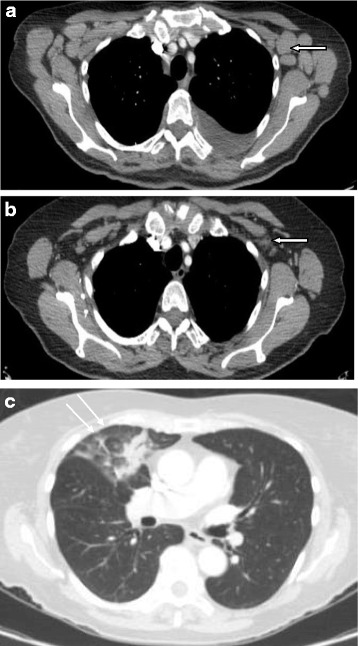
A 68-year-old female with CLL with 13q deletion on watchful waiting with progression of CLL a Axial CT of the chest obtained in arterial phase demonstrates bilateral axillary lymphadenopathy with the largest left axillary lymph node measuring 2 cm in short axis (white arrow). b Axial CT of the chest obtained 3 months after initiating Ibrutinib therapy reveals significant decrease in the lymphadenopathy with the axillary node now measuring less than a centimeter in short axis (white arrow). c Axial CT of the chest obtained in arterial phase as a part of restaging examination in the same patient reveals focal ground glass opacity in the right upper lobe (double arrows) raising concern for Ibrutinib pneumonitis
